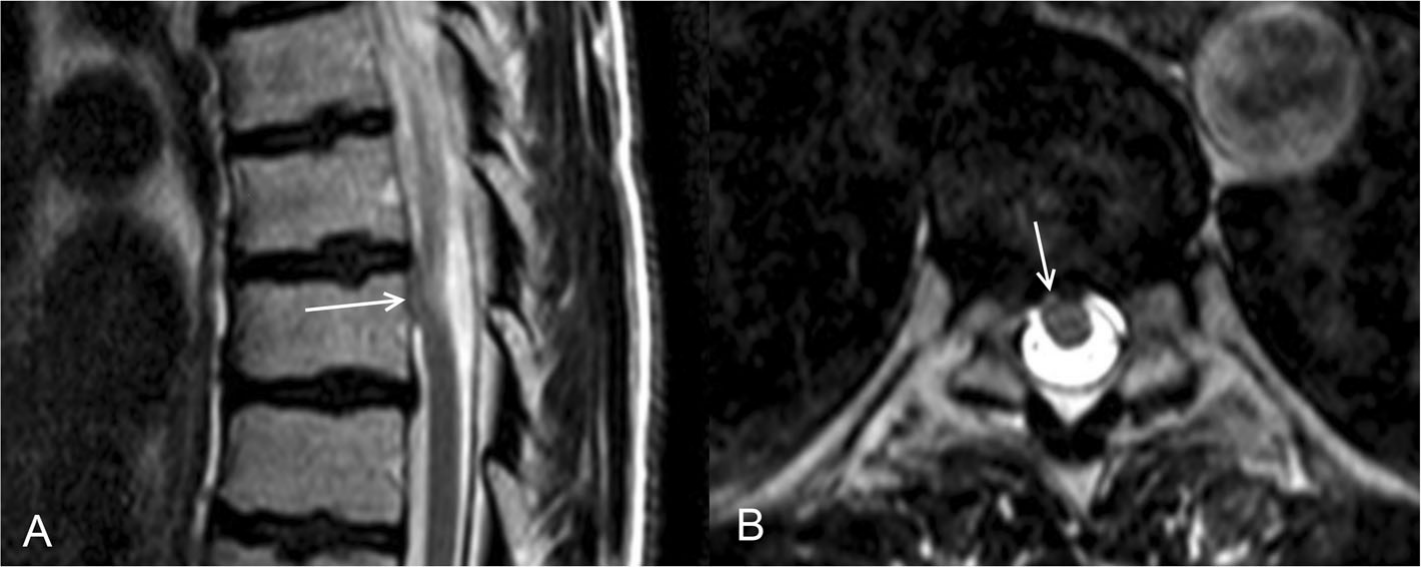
a Sagittal T2 weighted MRI of patient 2 showing a ventral displacement of the spinal cord due to spinal dysraphism with a hamartomatous growth (arrow). On the axial view (b), the defect is also shown (arrow) as a spinal dysraphism with a hamartomatous growth
